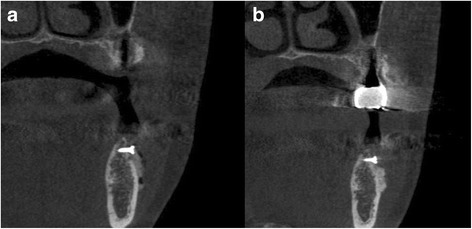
CBCT, postoperative view. a Immediately after the bone graft. b Four months after the graft
